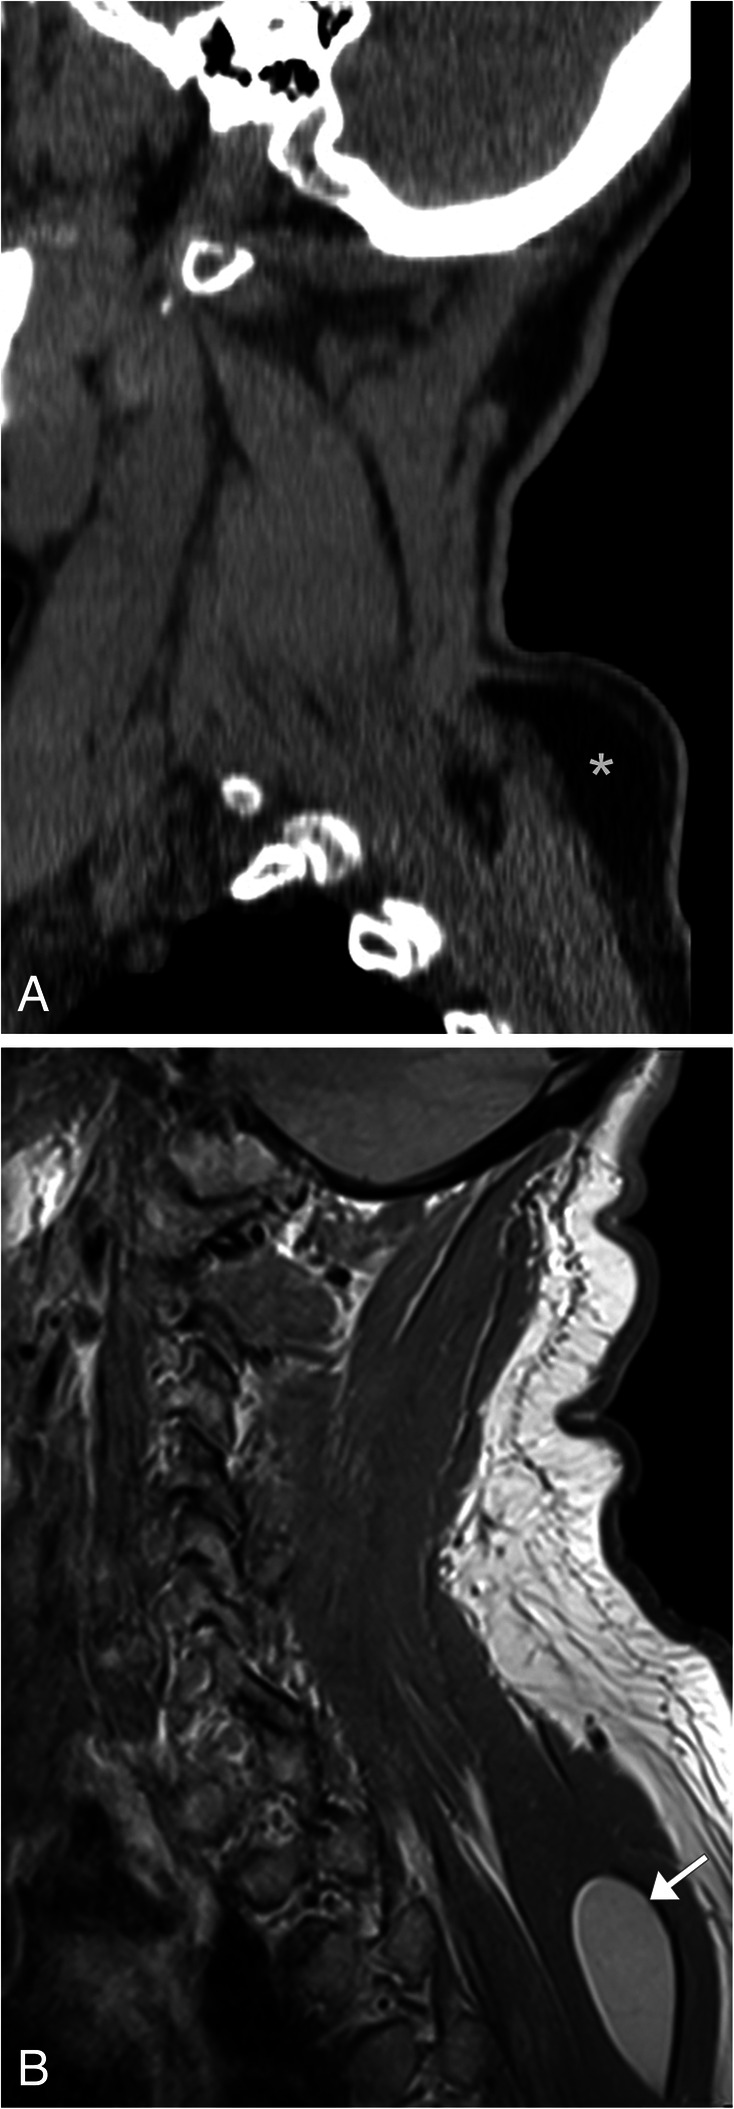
Lipoma. a Sagittal CT image of a companion case demonstrating a low-density lesion (*) measuring between − 65 and − 120 Hounsfield units in the posterior superficial soft tissue of the neck at the level of C7–T1. b Sagittal T1WI MR of the cervical spine shows a hyperintense lesion located between the posterior spinal muscles at the level of the upper thoracic spine (arrow), similar in signal to the subcutaneous fat
